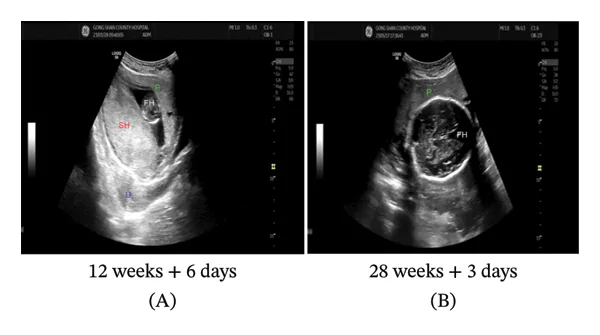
Comparison of ultrasound images before and after treatment. (A) Ultrasound images obtained at the initial diagnosis at 12 weeks and 6 days of gestation. A well‐demarcated, hypoechoic zone measuring 109 × 41 mm^2^, representing the largest cross‐sectional area, was identified beneath the gestational sac chorionic plate. Fetal examination is normal. (B) Ultrasound image at 28 weeks and 3 days of gestation after treatment. Subchorionic placental hematoma has resolved, placental echogenicity is normal, with no residual significant retroplacental or preplacental fluid collection detected, and both the fetus and amniotic fluid are normal. P represents the placenta; SH represents subchorionic hematoma; U represents the uterus; FH represents the fetal head.
